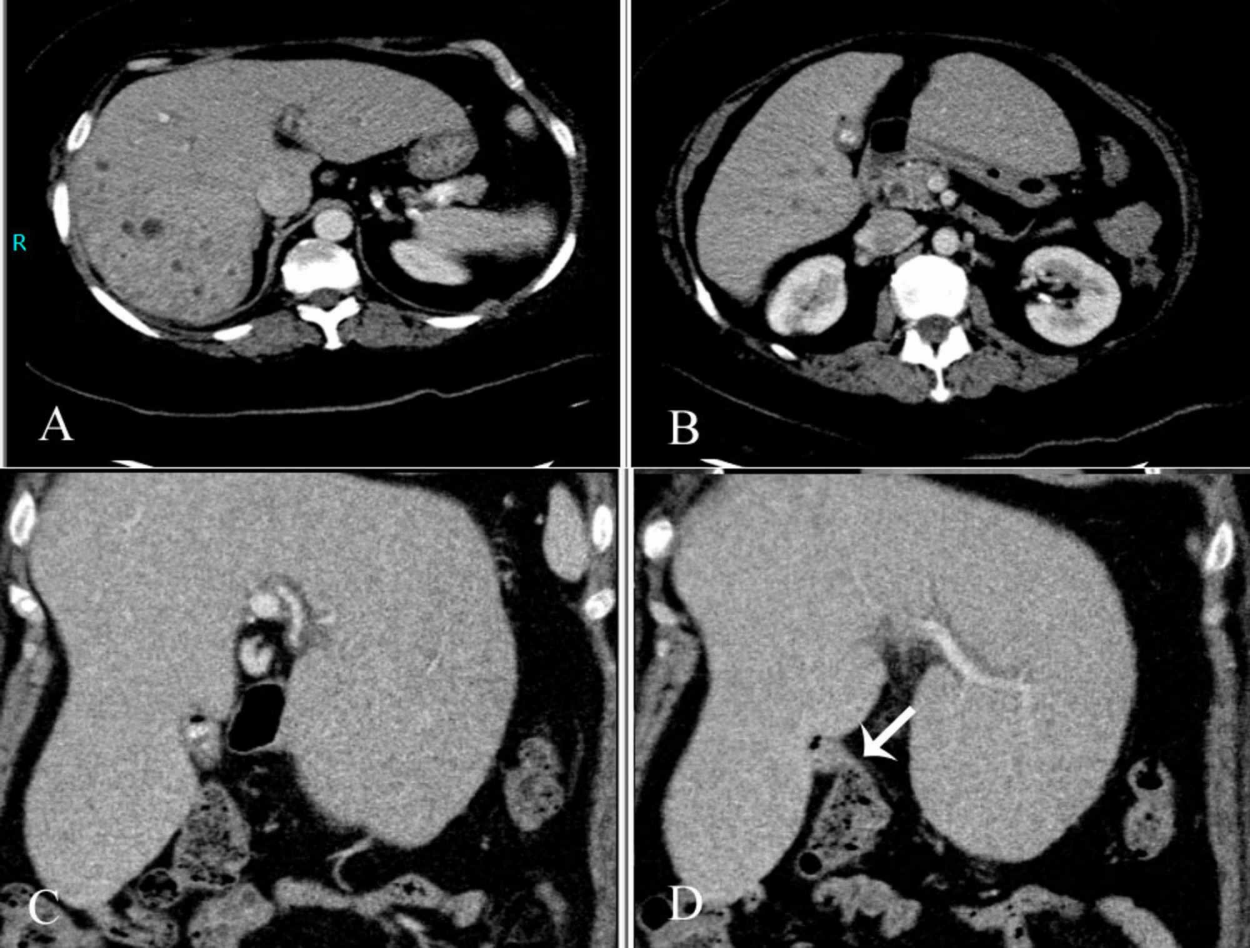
Computed tomography images A & B) Numerous cholangitic abscesses were seen in the right lobe of liver with contracted gallbladder containing calculi and few intraluminal air specks. C & D) Close approximation of hepatic flexure of colon with the gallbladder suggesting a fistulous communication (arrow).
